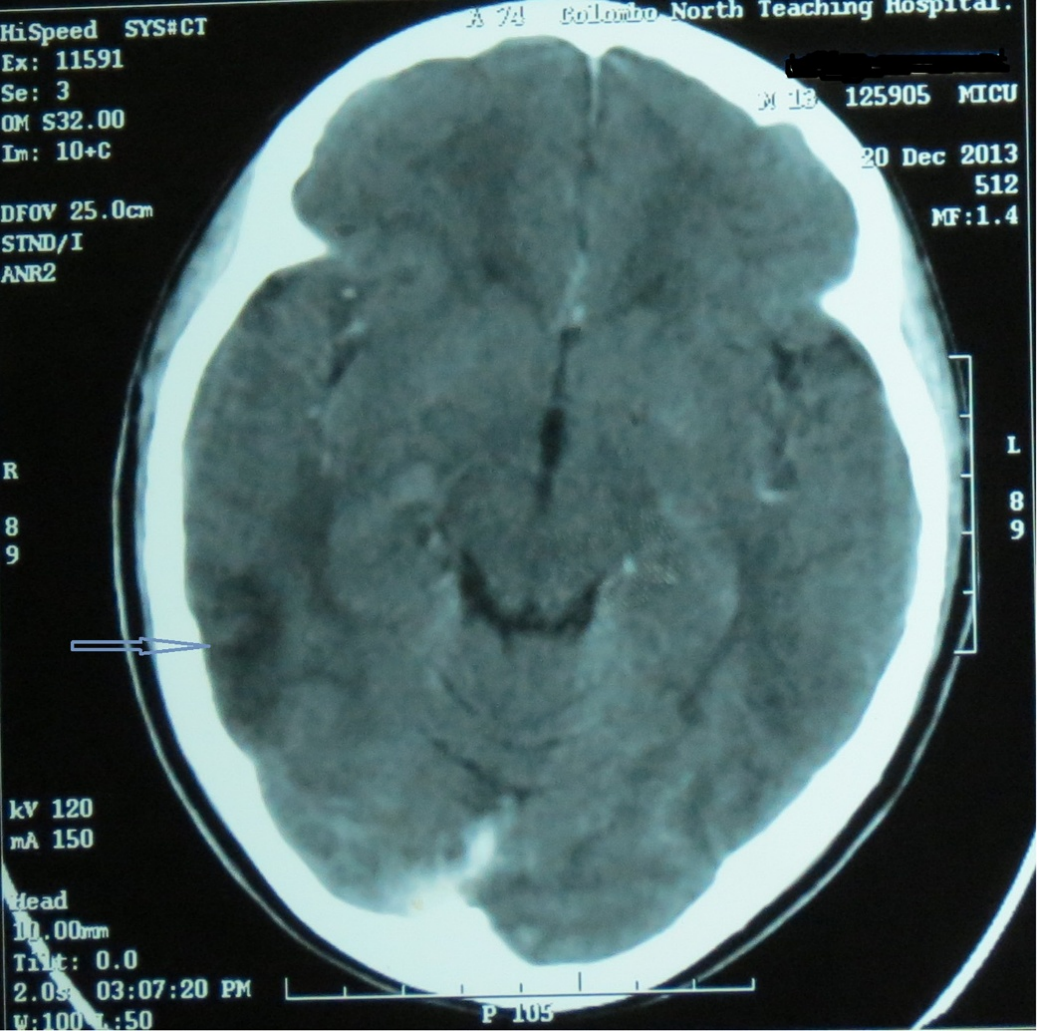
A contrast-enhanced computed tomography scan of brain done on day-3 showed non enhancing low attenuating areas in fronto -parietal regions (arrow).
